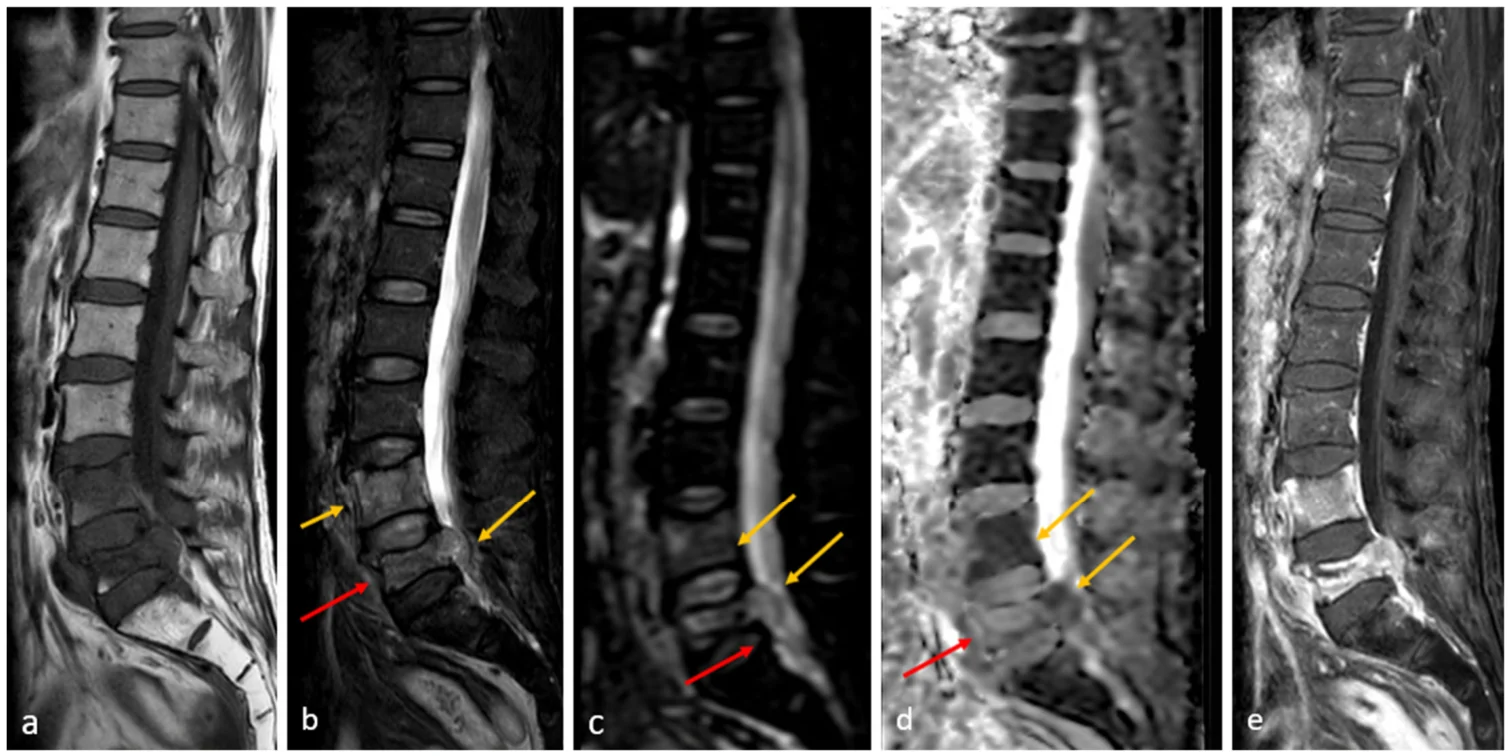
Diffusion-weighted imaging in the differentiation of benign from pathological vertebral compression fractures (imaging appearance in appropriate clinical context). The L4 vertebra and the posterior cortex of the L5 vertebral body (yellow arrows) demonstrate true restricted diffusion—hyperintense on T2-weighted (b) and DWI (c) images with corresponding hypointensity on the ADC map (d)—consistent with metastatic infiltration. In contrast, the L5 vertebral body, which shows the compression fracture, exhibits T2 shine-through (red arrows): hyperintensity on T2-weighted, DWI, and ADC images, attributable to vasogenic oedema. All these lesions appear hypointense on T1 (a), and post-contrast imaging (e) shows similar enhancement in both the metastatic lesions and the region of vasogenic oedema and is therefore of limited value in distinguishing the two.
